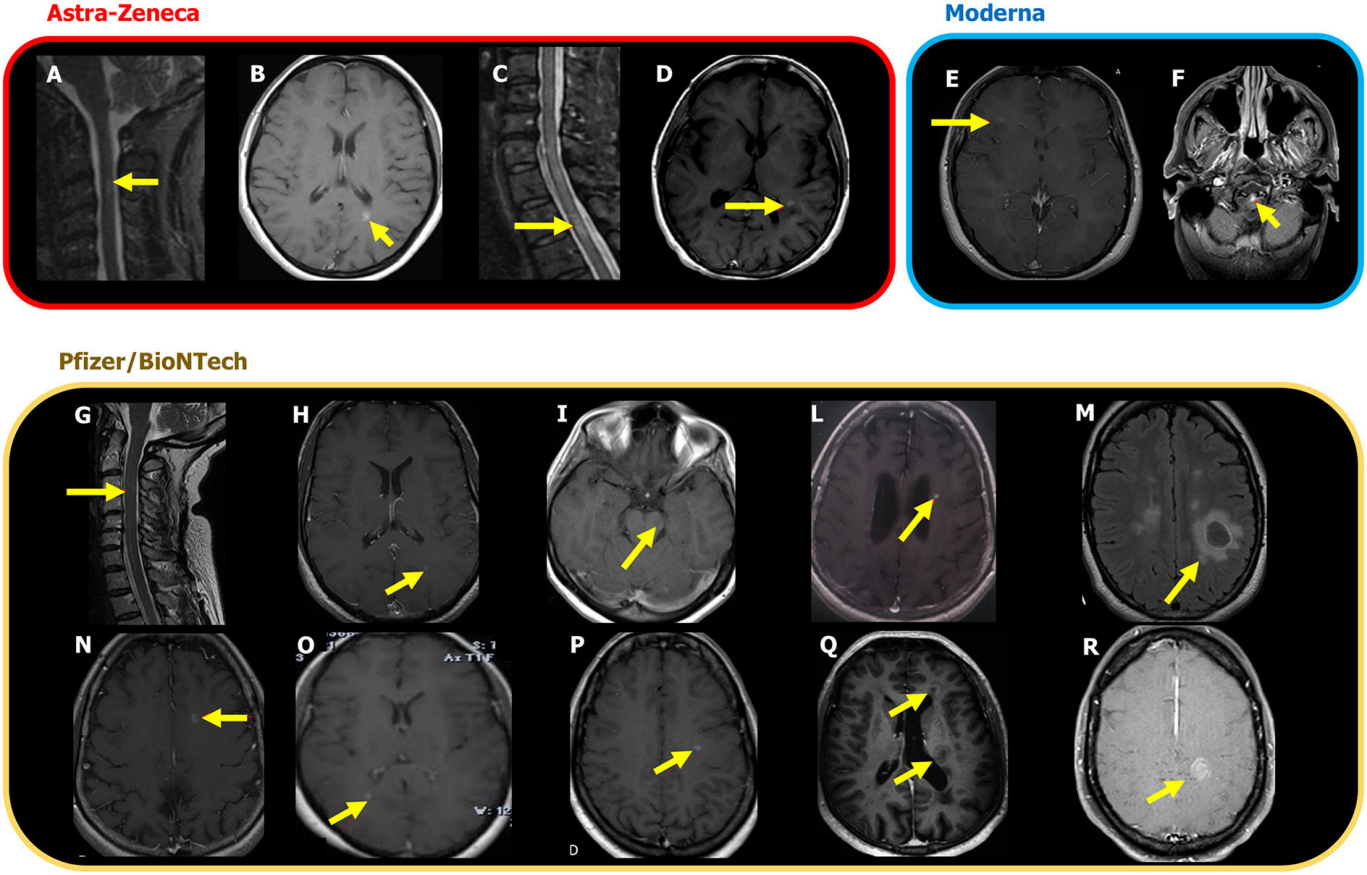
New MRI lesions associated with the MS episodes occurred after ChAdOx1 nCoV-19 (AZD12222), mRNA-1273, Moderna and BNT162b2, Pfizer/BioNTech vaccine. The lesions are shown on T2 weighted images or on post-contrast T1 weighted images and are indicated by yellow arrows. (A) Case 1: C3 lesion; (B) Case 2: new enhancing lesion in corpus callosum and multiple white matter unenhanced lesions in periventricular areas and in the mesial occipital lobe; (C) Case 3: new enhancing lesion in the thoracic cord; (D) Case 4: multiple hyperintense lesions in the supra and infratentorial white matter, four of which are with contrast enhancement; (E) Case 5: new brain lesion with contrast enhancement. (F) Case 6: enhancing bulbar lesion; (G) Case 7: C3 lesion with contrast enhancement; (H) Case 8: new brain enhancing lesion; (I) Case 9: a new contrast enhancing lesion in the mesencephalon. (L) Case 10: new enhancing brain lesion. (M) Case 11: enhancing brain lesion with conspicuous oedema; (N) Case 12: a new active lesion with ring enhancement in the left frontal white matter. (O) Case 13: three new brain enhancing lesions, one of which is indicated by the arrow; (P) Case 14: three new enhancing lesions in the left temporal lobe and one, indicated here, in the left centrum semiovale; (Q) Case 15: two ring-enhancing lesions localized in the white matter adjacent to the left frontal horn and in the left middle periventricular region. (R). Case 16: new enhancing lesions, one of which is tumefactive, localized in the white matter of the left centrum semiovale.
